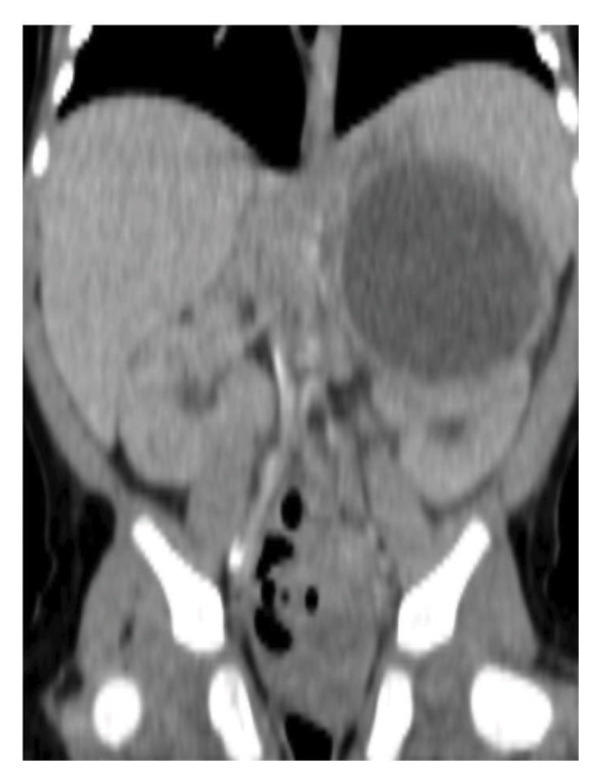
Contrast‐enhanced CT scan (Day 36) showing a cystic suprarenal lesion with heterogeneous internal contents, without enhancement or calcifications, raising suspicion of infected hematoma versus adrenal abscess.
